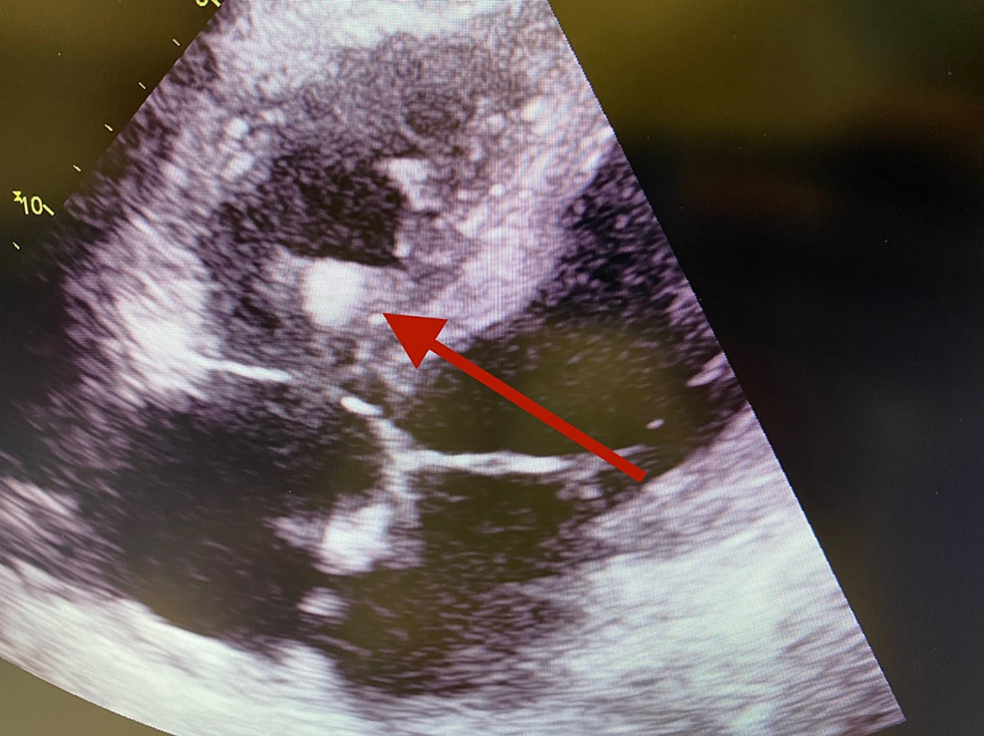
Echocardiogram showing intracardiac thrombus in right ventricle on subcostal view
